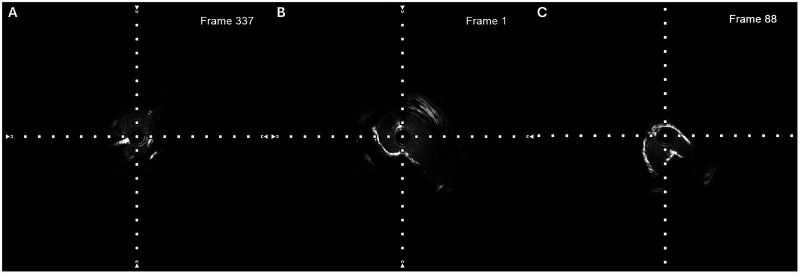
Intravascular ultrasound (IVUS) images from the left main (LM) coronary artery. (A) Ostial LM severe stenosis with a minimal lumen area of 5.5 mm². (B) Ostial LCx segment with marked plaque burden. (C) LM bifurcation showing heavily calcified stenosis with a protruding calcified nodule.
